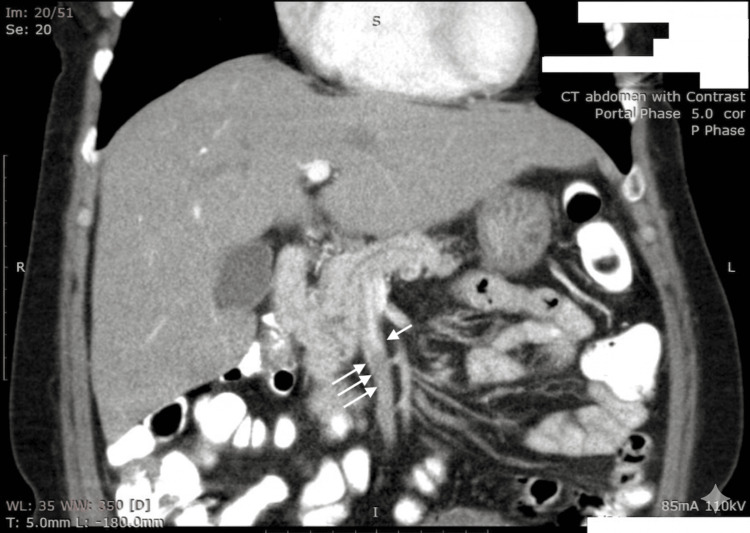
Contrast-enhanced CT abdomen (portal venous phase) The image shows filling defects in the middle and distal portions of the SMV (arrows), compared with the normal contrast enhancement of the proximal SMV (arrow) CT: computed tomography; SMV: superior mesenteric vein
